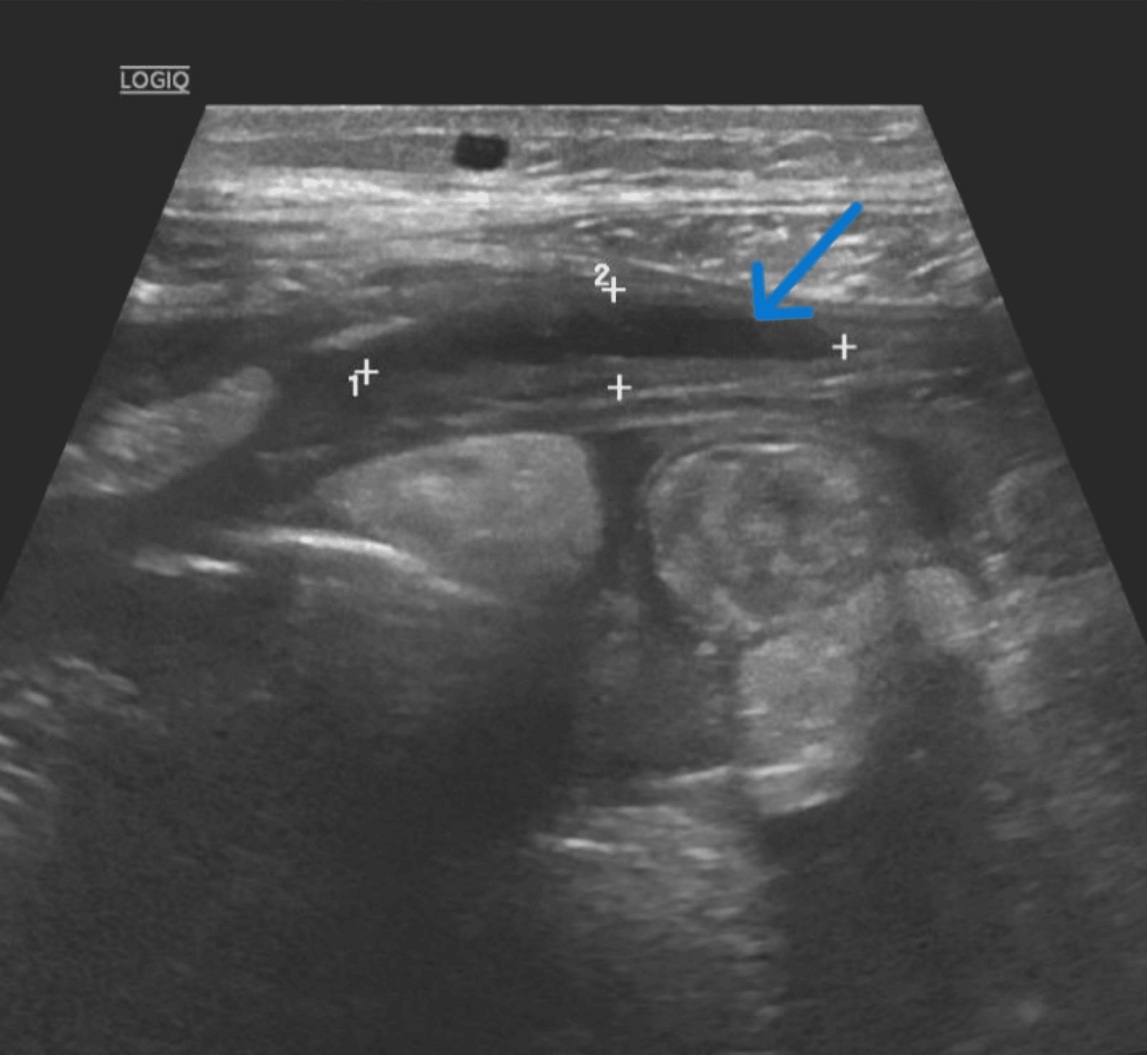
Abdominal ultrasound at the end of the patient's hospital course post-tPA dwells. Ultrasound demonstrates significantly improved fluid collection in his right abdomen without apparent loculations and partially imaged drain. tPA: alteplase
